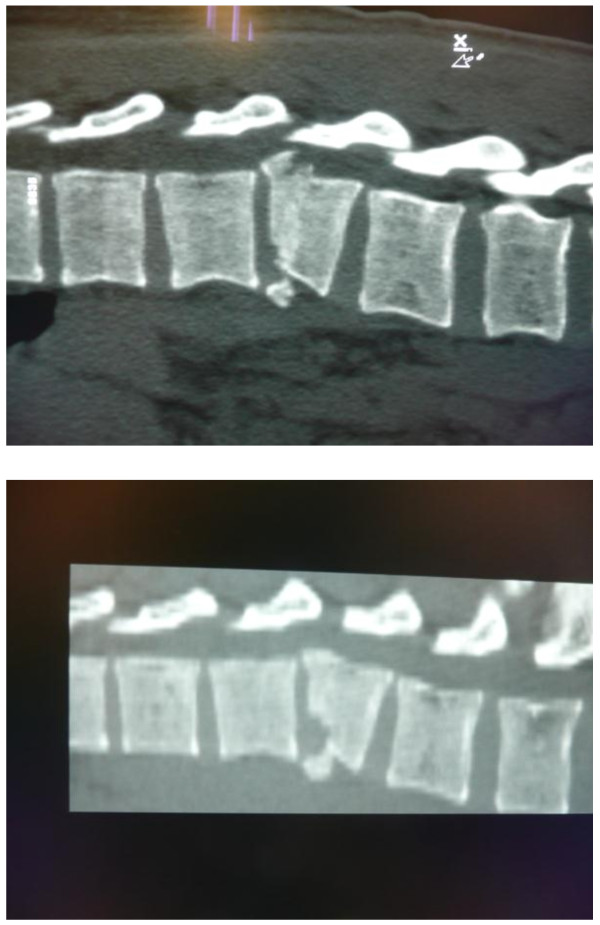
Case pair A, two sagittal reconstructions of CT-scans of two separate thoracic vertebral columns of two passengers of an armored personnel carrier that hit an improvised explosive device (IED). Both showed identical, unstable burst-split fractures of the 9^th^ thoracic vertebra.
